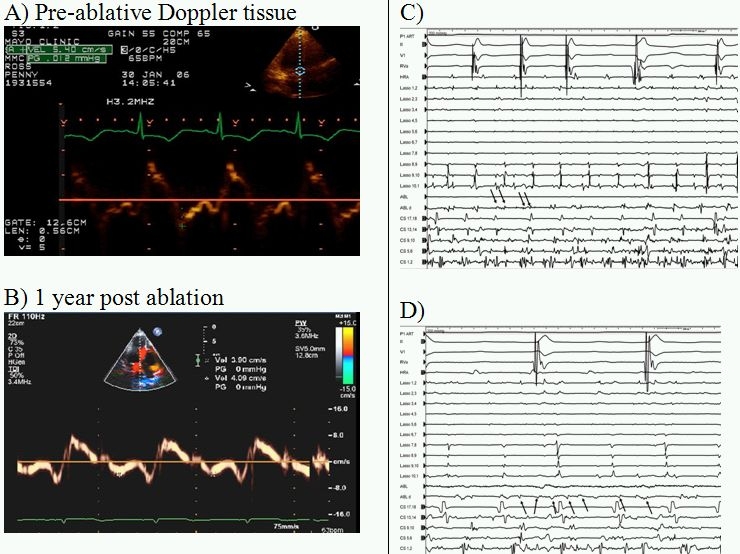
A pre-ablation Doppler velocity tracing showing moderate diastolic dysfunction (e' cm/s).  Echo parameters were essentially unchanged 1 year post ablation B (e' 4 cm/s and LAVI 72 ml/m^2^).  C/D show pre- and post-ablation electrograms with varying degrees of organization during persistent fibrillation and highly fragmented signals that correlated with echo parameters signifying advanced secondary atrial disease.
